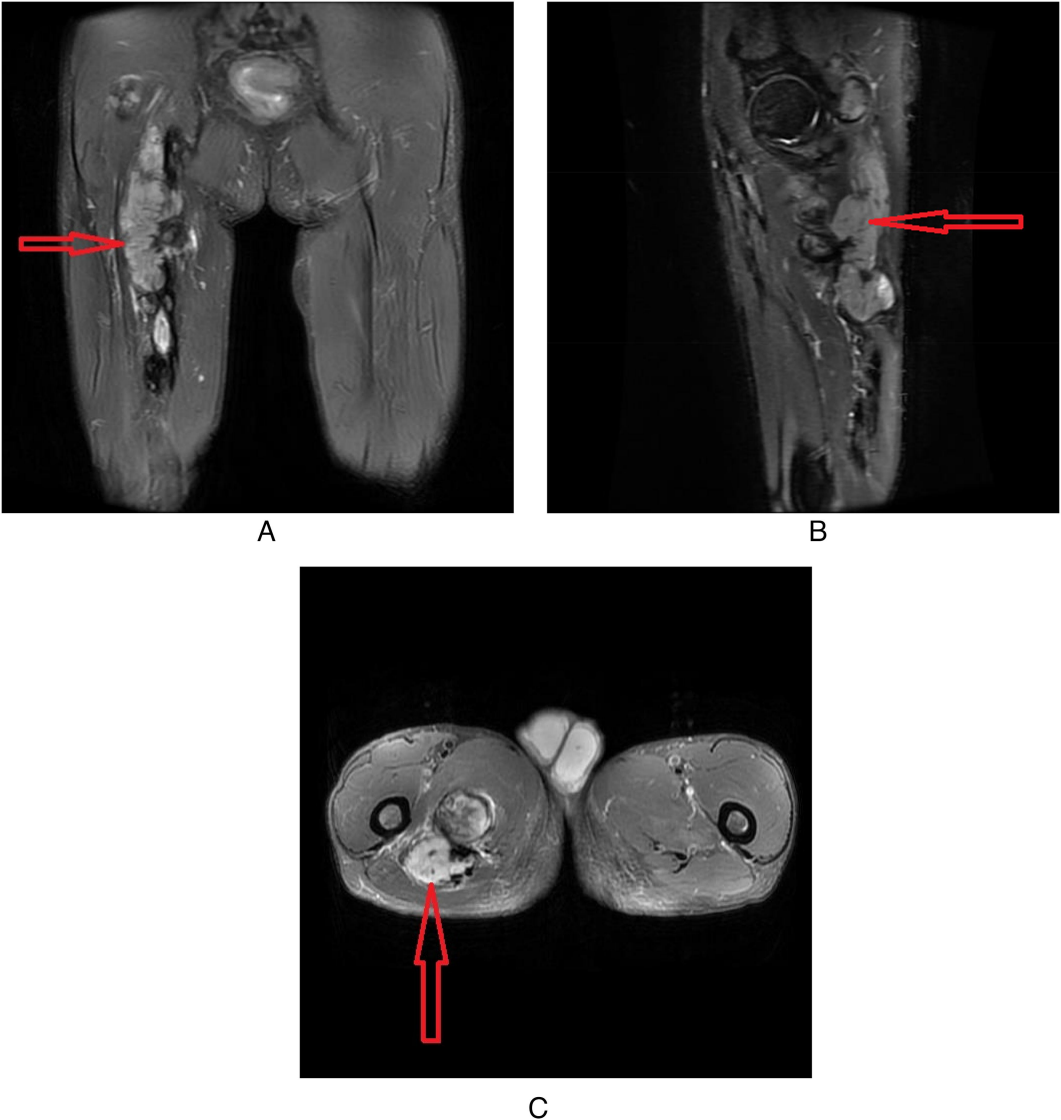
(A) MRI features of the tumor in the coronal plane. (B) MRI features of the tumor in the sagittal plane. (C) MRI features of the tumor in the axial plane.
